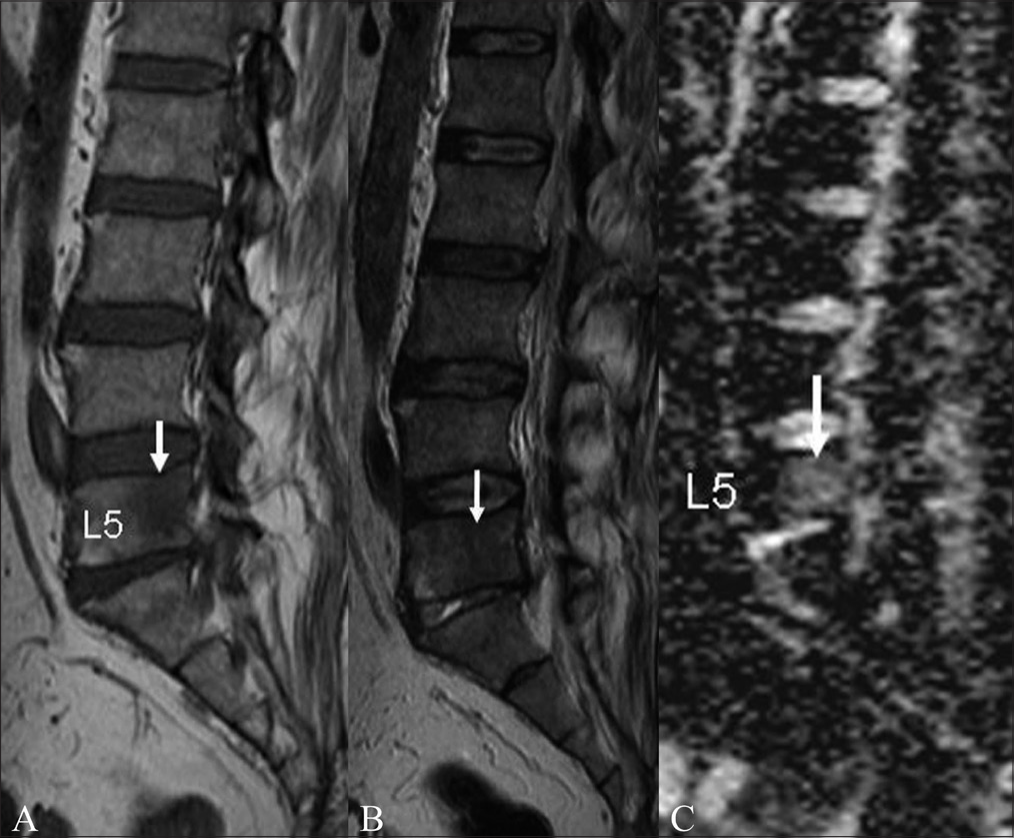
Tuberculosis of spine. Sagittal T1W (A) and T2W (B) images in a 40-year-old patient with spinal tuberculosis show a well-defined rounded lesion (arrow) with T1 hypointensity and T2 iso-hypointensity, which was difficult to characterize on routine MRI imaging. Sagittal ADC map (C) reveals increased diffusion in the lesion with an ADC value of 1.46 × 10^−3^ mm^2^/s
